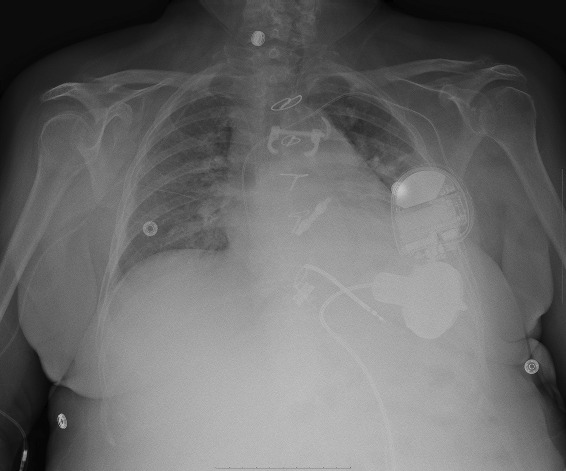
Chest X-ray on Day 28 showing decreased pulmonary opacities.
